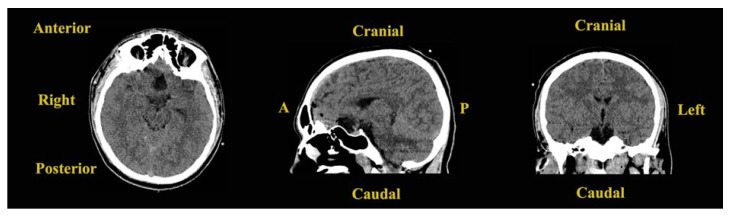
Postoperative native brain CT scan shows a normal aspect (A-anterior; P-posterior).
